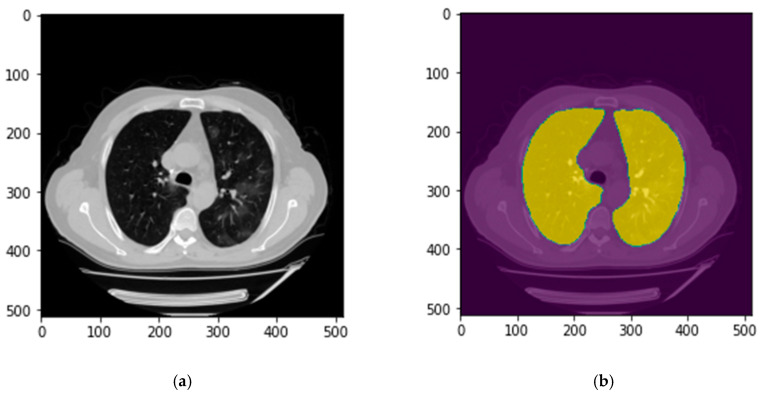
An example of the lung segmentation. (a) Raw CT image; (b) CT image and lung mask.
